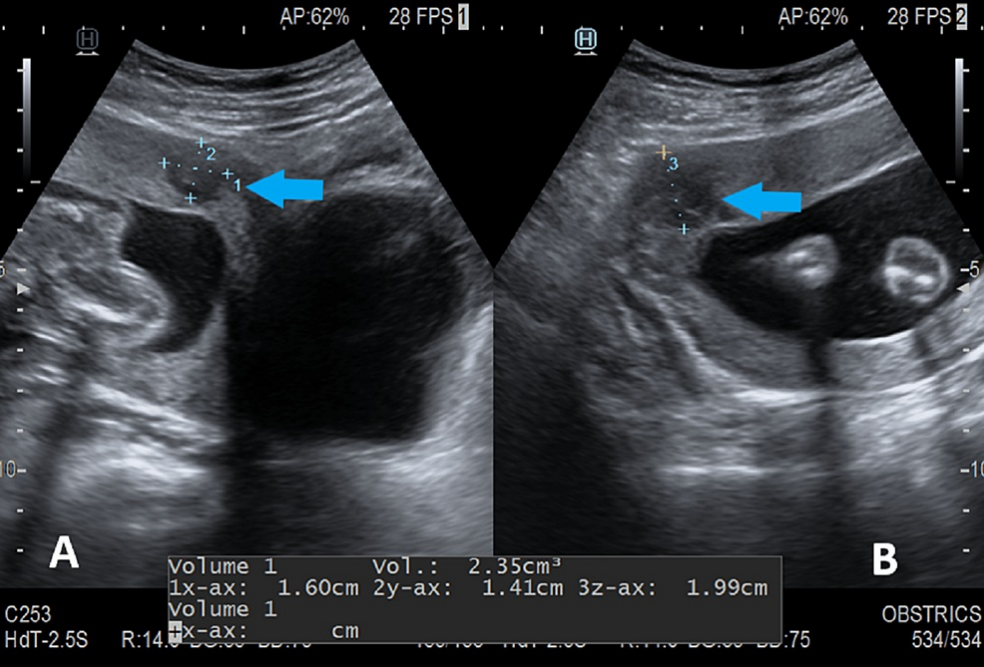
Transabdominal ultrasound sagittal (A) and axial (B) sections show minimal placental separation with a small retroplacental collection measuring approximately 2.35cc (blue arrow) suggesting retroplacental hemorrhage.
